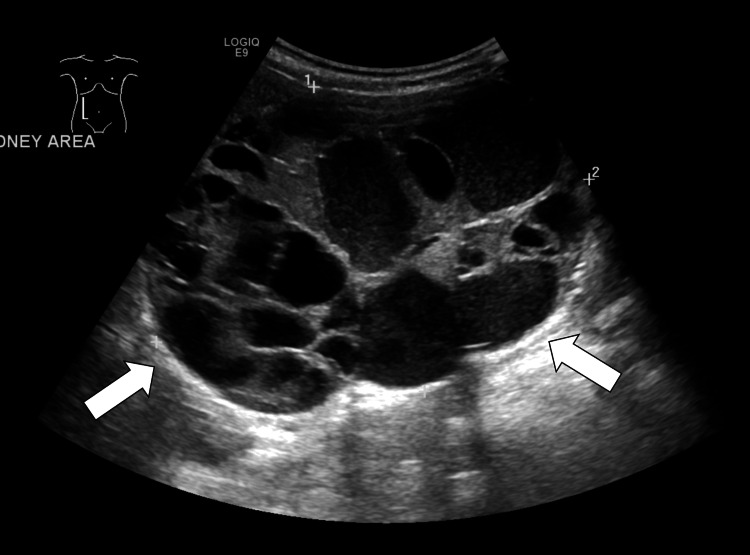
Ultrasound scan over the right kidney shows multiple cystic lesions with multiple loculations with no evidence of discernible solid component (arrows)
